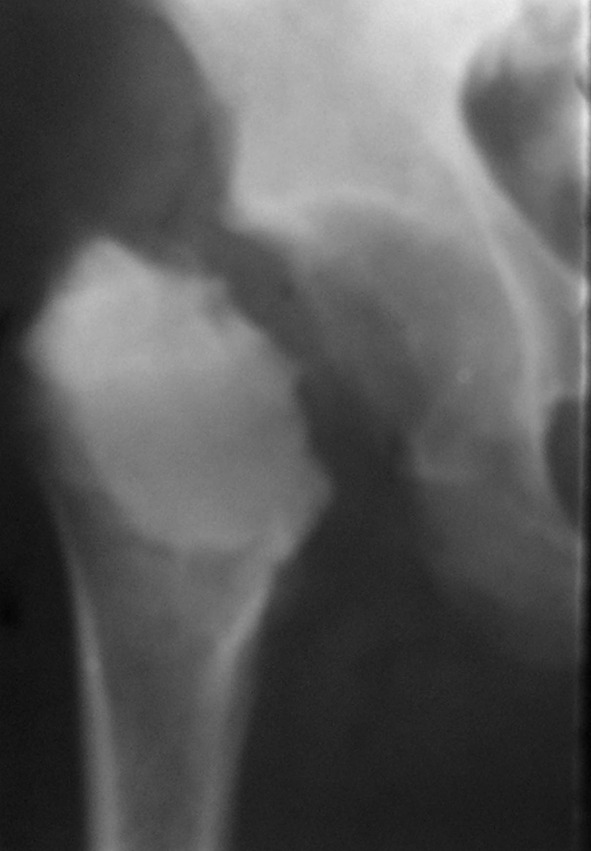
Radiograph of a 40-year-old female (case 6) showing Girdlestone resection arthroplasty with cementation seen in an osteolytic lesion
